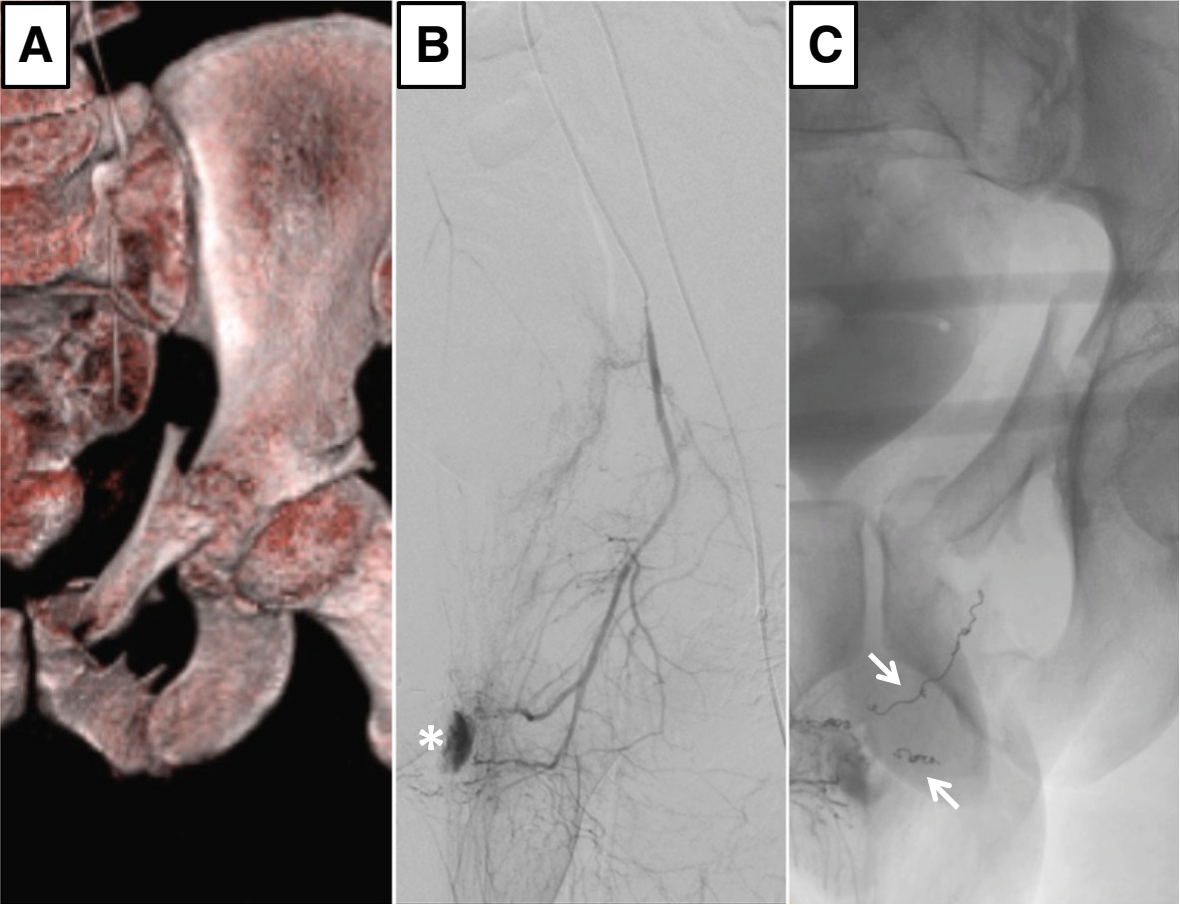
Pelvic fracture with associated arterial bleeding and consecutive embolization. The figure shows a 3D-reconstruction of a pelvic CT, with a type C pelvic fracture and destruction of the superior and inferior pubic rami at the time of trauma room admission in a hemodynamic unstable patient (a). CTA was suggestive of pelvic arterial bleeding and immediate angiography was indicated. Digital subtraction angiography (DSA) (b) revealed arterial bleeding from branches of the obturator artery with pooling of contrast agent around the symphysis (*). Super-selective microcoil embolization (arrows) of the feeding vessels helped to control the bleeding and stabilize the patient (c)
